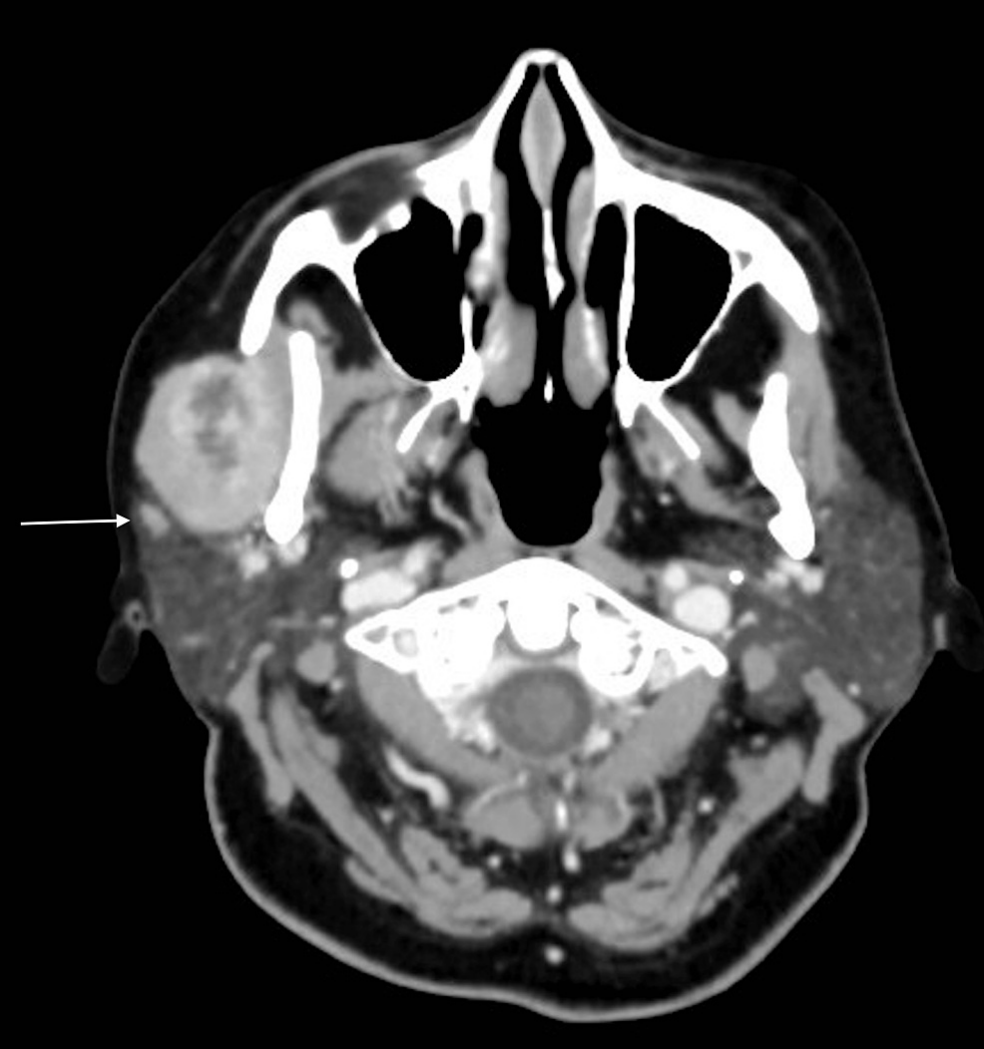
Preoperative neck CT scan A neck CT scan showed a well-circumscribed heterogeneously enhancing lesion with central hypodensity measuring 3X2.7X3.5 cm. The white arrow is pointing to the retromandibular vein.
